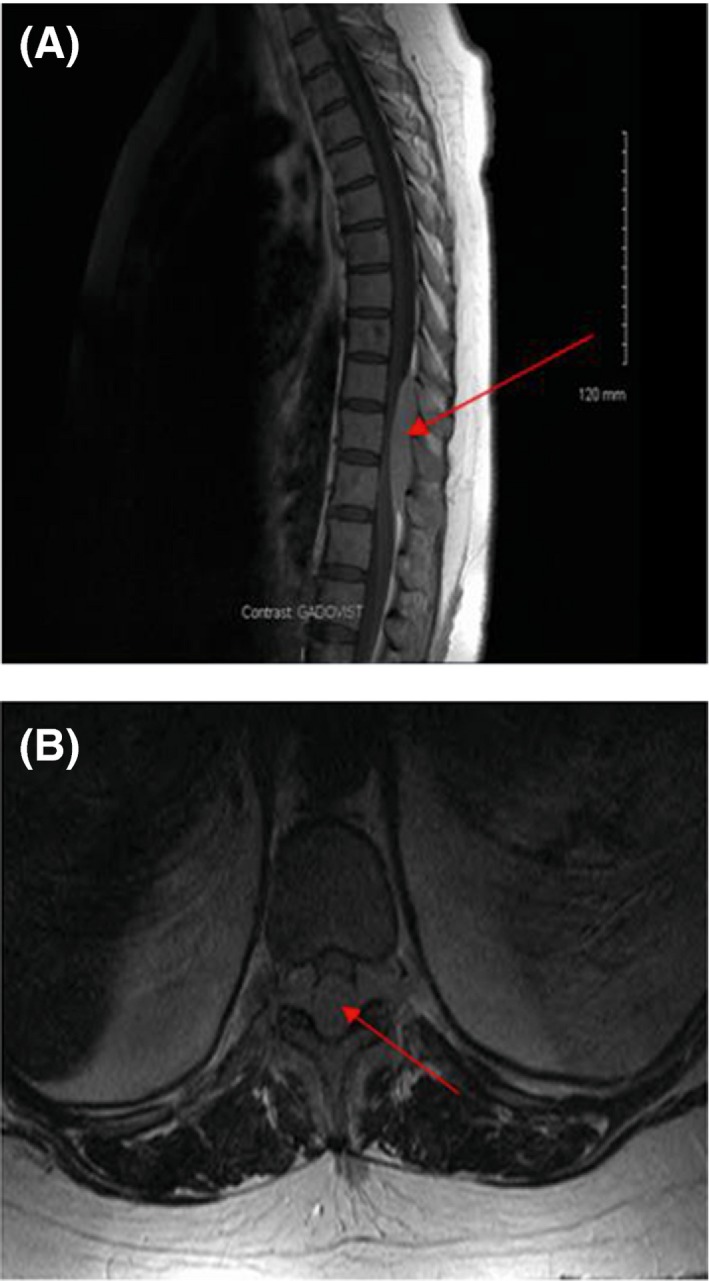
MRI thoracic spine showing posterior extradural extramedullary neoplastic mass (red arrows), extending from T10 to T12 (A, sagittal view; B, axial view).
